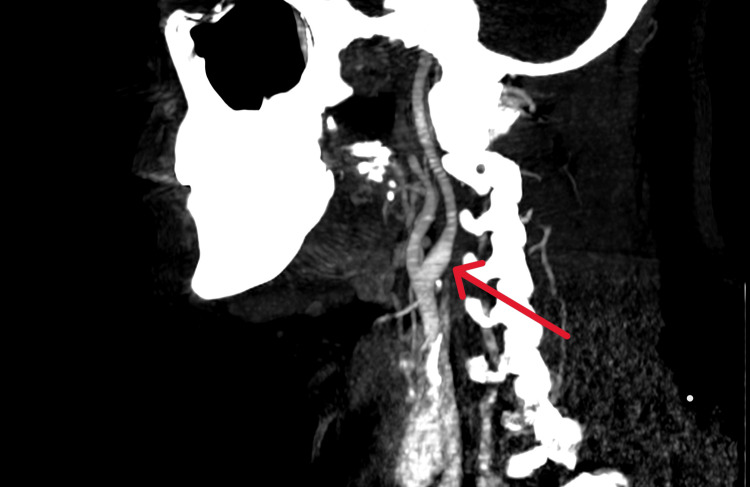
CT angiogram after one week of therapy showing subtle wall thickening in the left ICA without any visible filling defect (red arrow) ICA: Internal carotid artery
